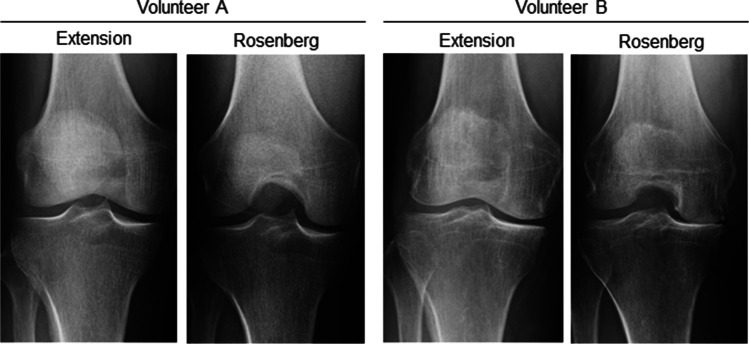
Knee radiographs of full extension and Rosenberg weight-bearing views. In volunteer A, the minimum joint space width (mJSW) at the medial compartment is similar between the full extension and the Rosenberg views. In volunteer B, the mJSW is narrower in the Rosenberg view than in the full extension view
